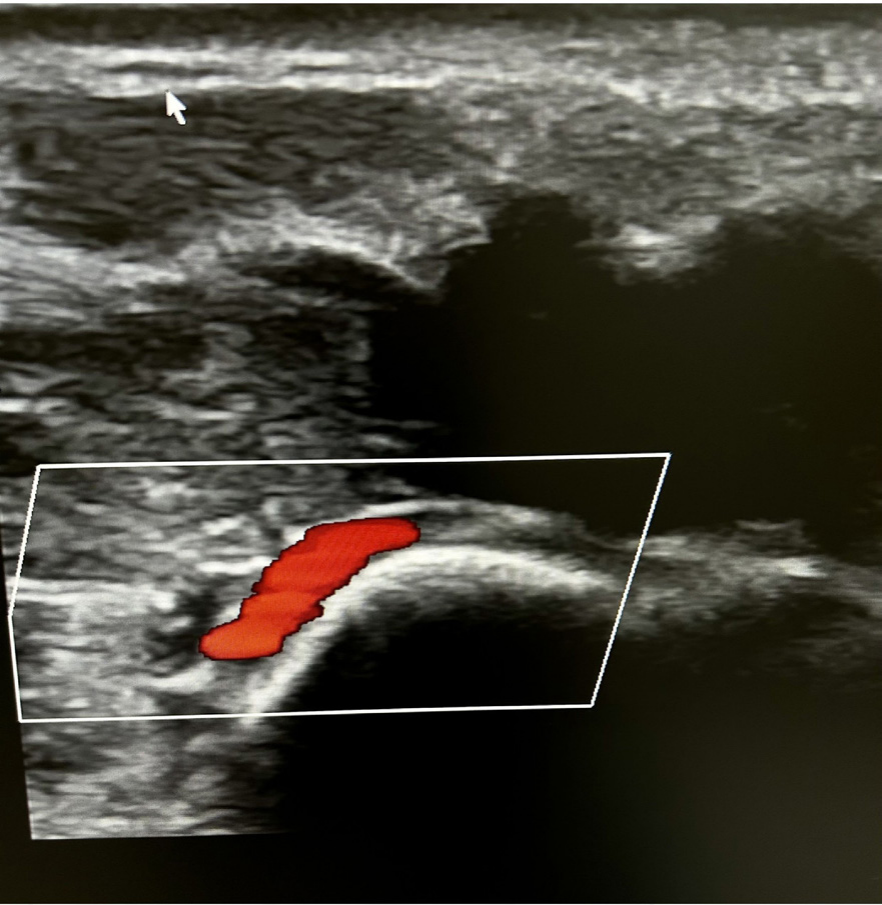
Deep palmar arch patent on color Doppler ultrasonography.
